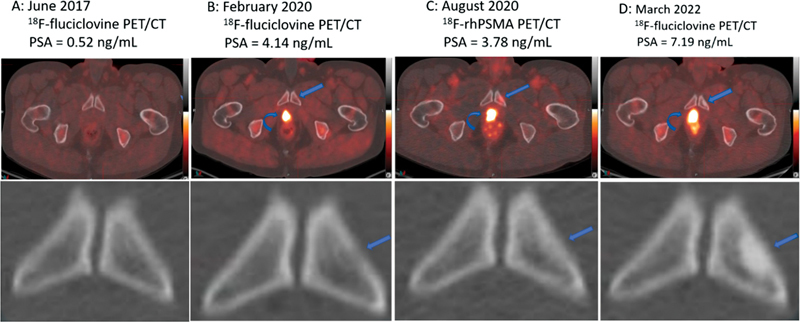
A 57-year-old man with prostate cancer recurrence had multiple time-point positron emission tomography/computed tomography (PET/CT) images showing the temporal evolution of untreated prostate cancer skeletal metastasis. (
